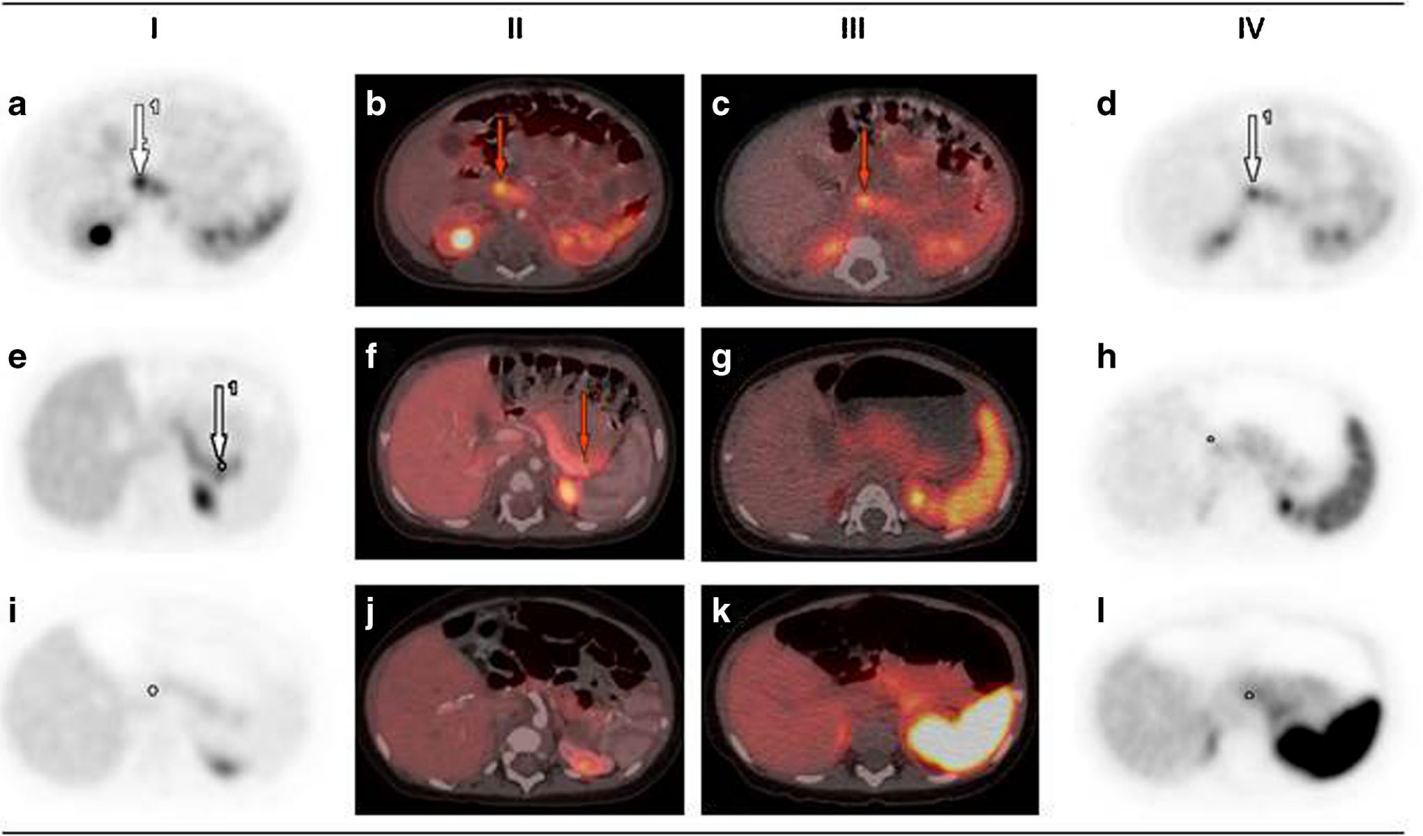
18F–DOPA PET/CT and 68Ga-DOTANOC PET/CT performed in three patients. a–d Patient 1, focal CHI. e–h Patient 2, focal CHI. i–l Patient 3, diffuse CHI. Column I shows 18F–DOPA PET; column II 18F–DOPA PET/CT (here contrast-enhanced); column III 68Ga-DOTANOC PET/CT; column IV 68Ga-DOTANOC PET. Red arrows point to the focal lesions. In the blinded evaluations, patient 1 and 2 were correctly diagnosed as focal and patient 3 as non-focal CHI by 18F–DOPA PET/CT (visual and by cut-off method). By 68Ga-DOTANOC PET/CT, patient 1 and patient 3 were correctly classified, but patient 2 was wrongly classified as non-focal
